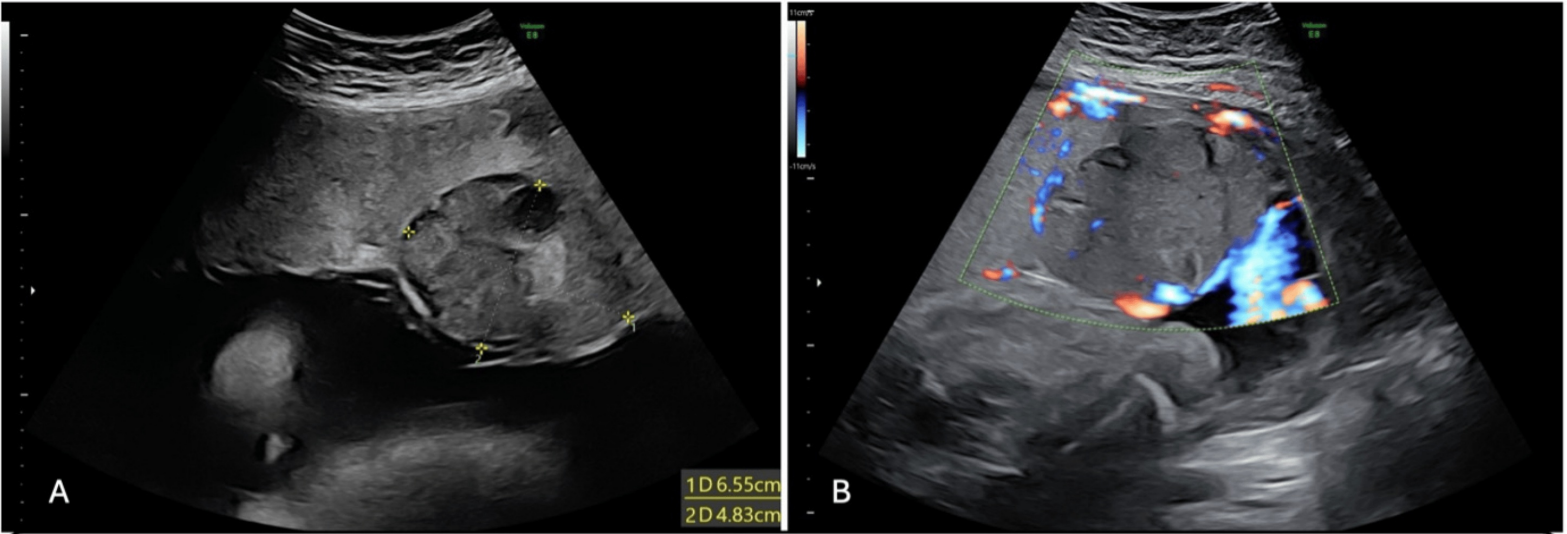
Ultrasound imaging A) Transabdominal obstetric ultrasound depicting gray-scale image of a chorioangioma. A well-defined mass measuring 65x48 mm with heterogenous echotexture observed in the placenta; B) Color doppler image of the chorioangioma revealed a highly vascularized placental tumor protruding into the amniotic cavity.
